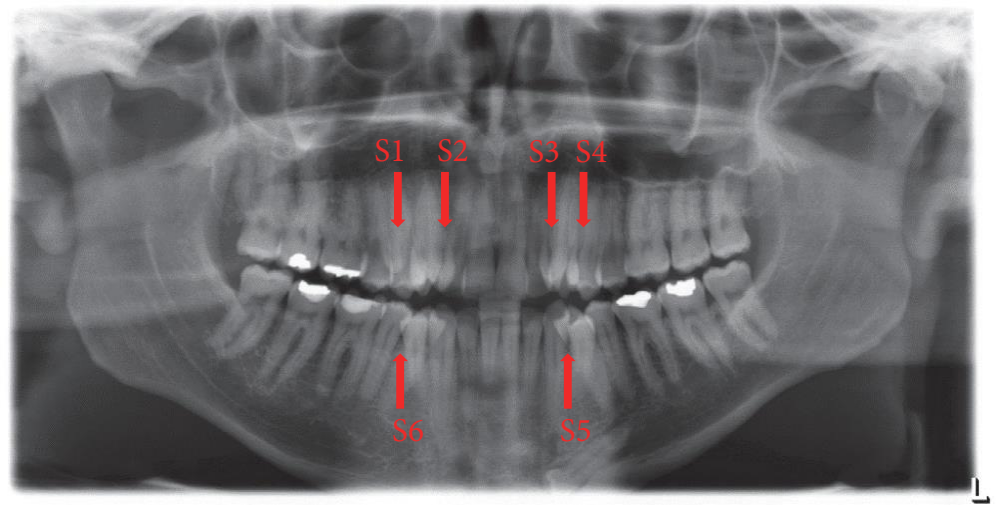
Orthopantomogram showing six supernumerary teeth in the maxillary premolars (S1, S4) and lateral incisors (S2, S3) area and mandibular premolar areas (S5, S6).
